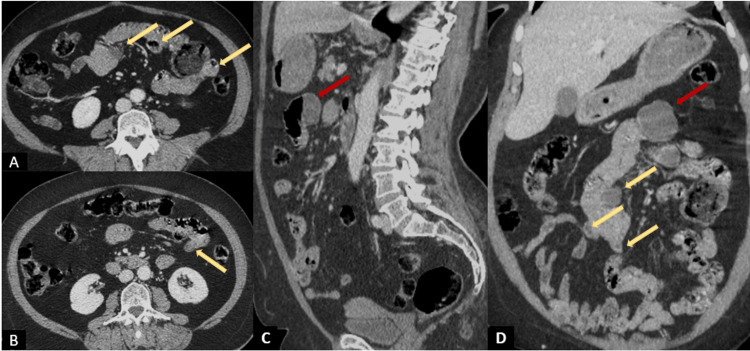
Contrast-enhanced abdominal CT (portal venous phase) with axial (A, B), sagittal (C), and coronal (D) reformations demonstrating multiple air-filled and air-fluid outpouchings arising from the jejunal wall (yellow arrows). The largest lesion is located in the proximal jejunum (red arrows).
